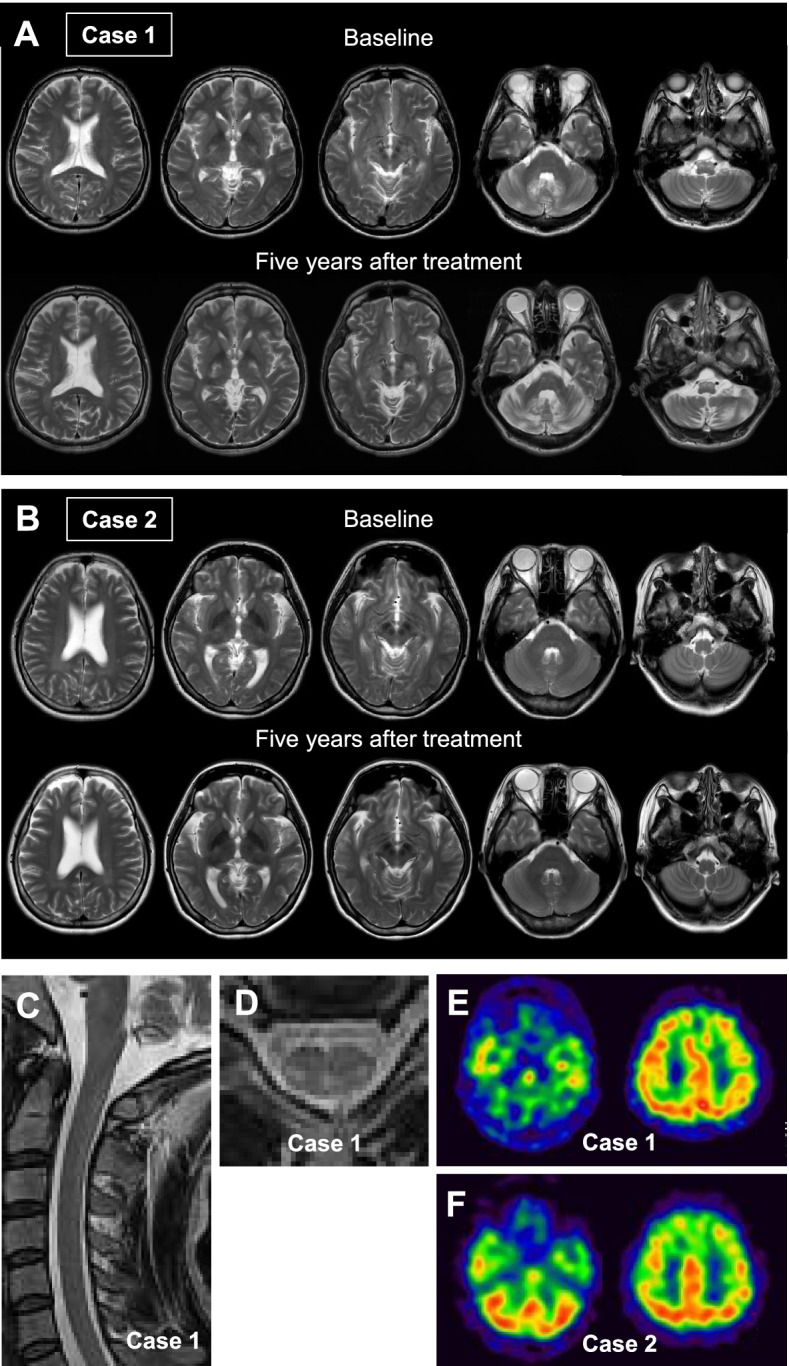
Baseline and 5-year follow-up brain magnetic resonance imaging (MRI) of Case 1 (A) and Case 2 (B). Axial T2-weighted images at baseline in Case 1 (A, upper panel) and Case 2 (B, upper panel). Five-year follow-up MRI in Case 1 (A, lower panel) and Case 2 (B, lower panel). T2-weighted spinal cord MRI at baseline in Case 1 (C, D). Cervical cord, sagittal view (C). Cervical cord, axial view at level C5 (D). Brain ^99^mTc-ethyl cysteinate dimer single photon emission computed tomography in Case 1 (E) and Case 2 (F)
